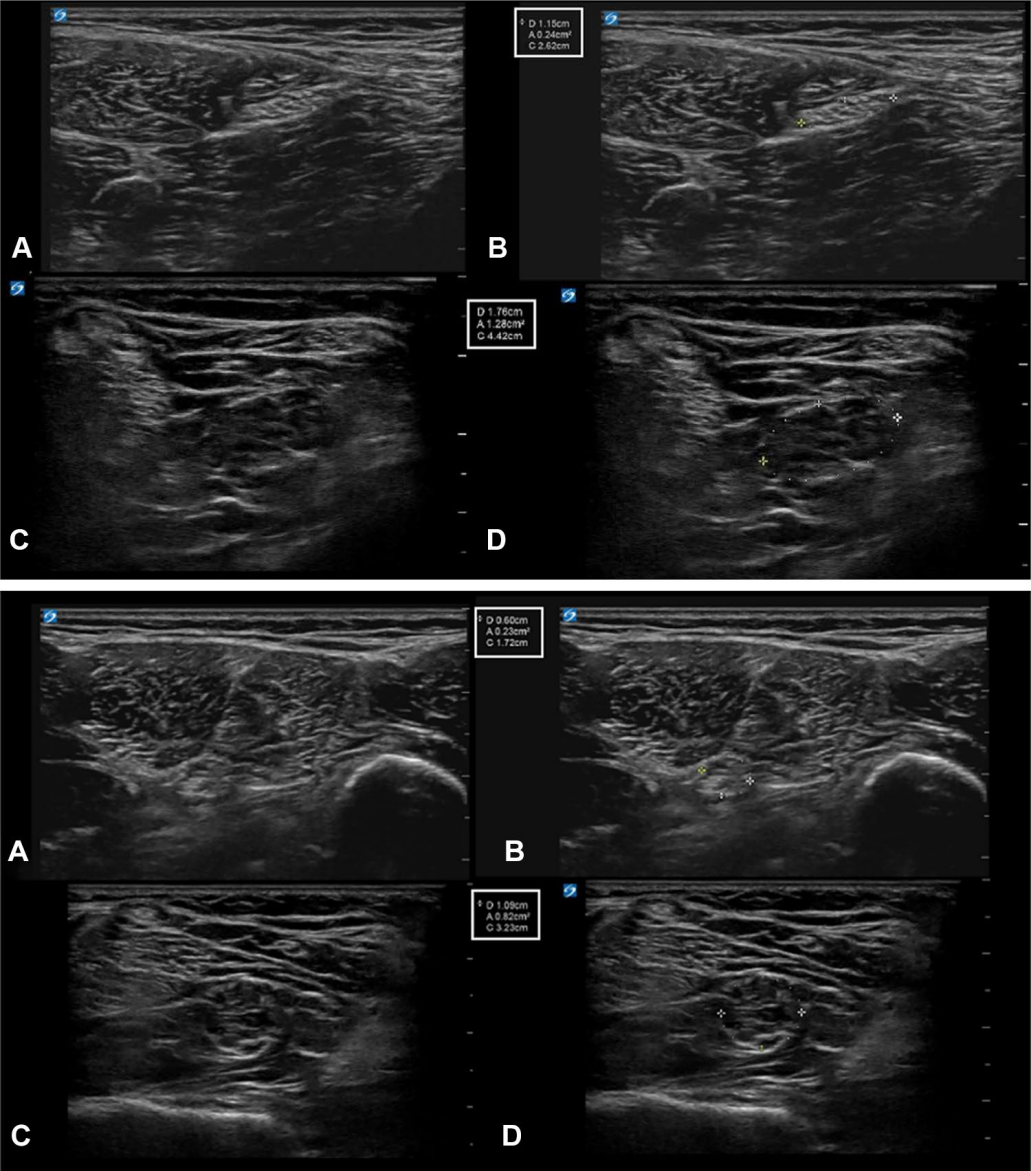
Nerve ultrasound images of patient 4 (C and D) compared to a control individual (A and B). Upper panel: the proximal sciatic nerves without (A and C) and with (B and D) cross-sectional areas. The normal nerve has a size of 24 mm^2^, which is a normal size for a 10-year-old child, while the enlarged nerve in the 10-year-old girl is 128 mm^2^. Lower panel: the distal sciatic nerves without (A and C) and with (B and D) cross-sectional areas. The normal nerve has a size of 23 mm^2^, which is a normal size for this 10-year-old child, while the enlarged nerve in a 10-year-old girl is 82 mm^2^
